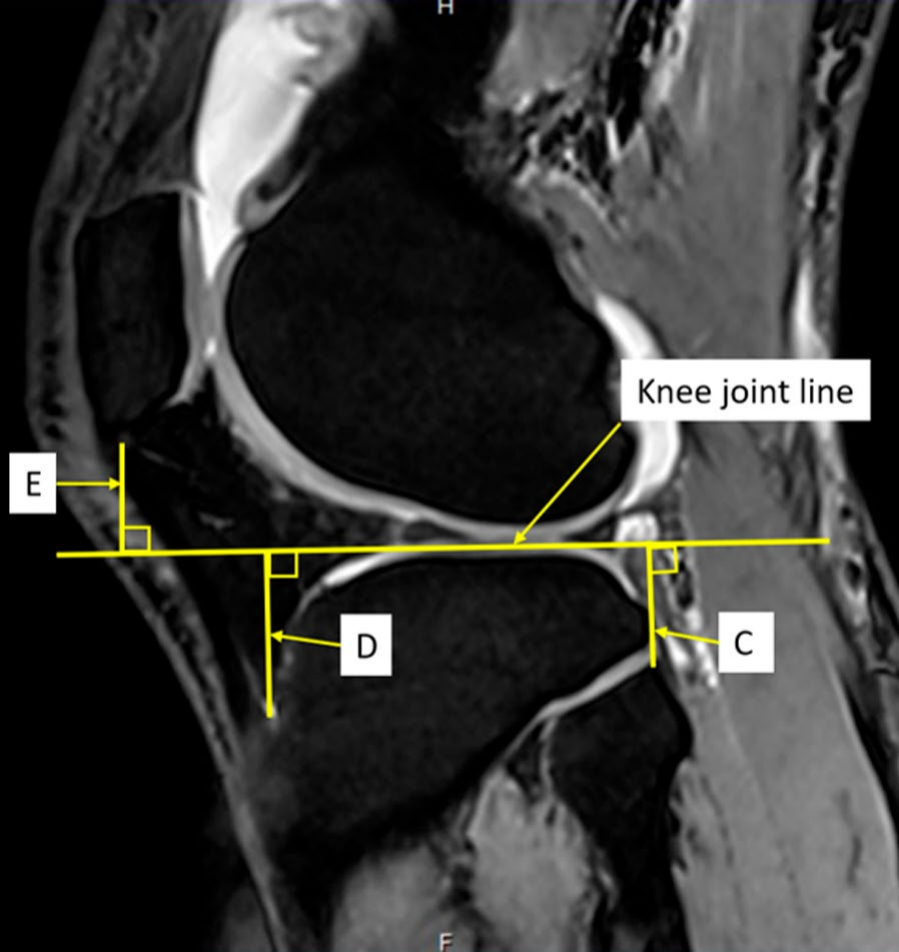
Demonstration of the measurement technique in sagittal view of MRI. The knee joint line was identified by the line between the highest points of cartilage of the anterior and the posterior tibial plateau. Line *C* is the distance from the highest point of fibular head perpendicular to the knee joint line. Line *D* is the distance from tibial tubercle to the knee joint line. It is measured from the most proximal point where the patella tendon is inserted into the tibial tubercle to the knee joint line. Line *E* is the distance from the most inferior point of inferior pole patella perpendicular to the knee joint line
